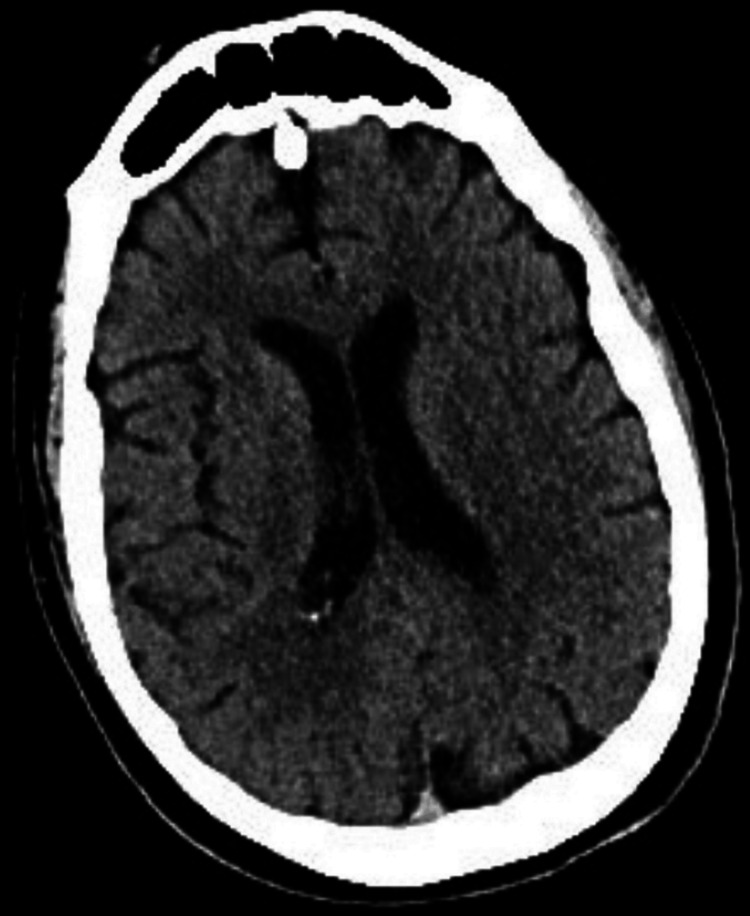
CT head obtained at admission revealed no acute intracranial process
